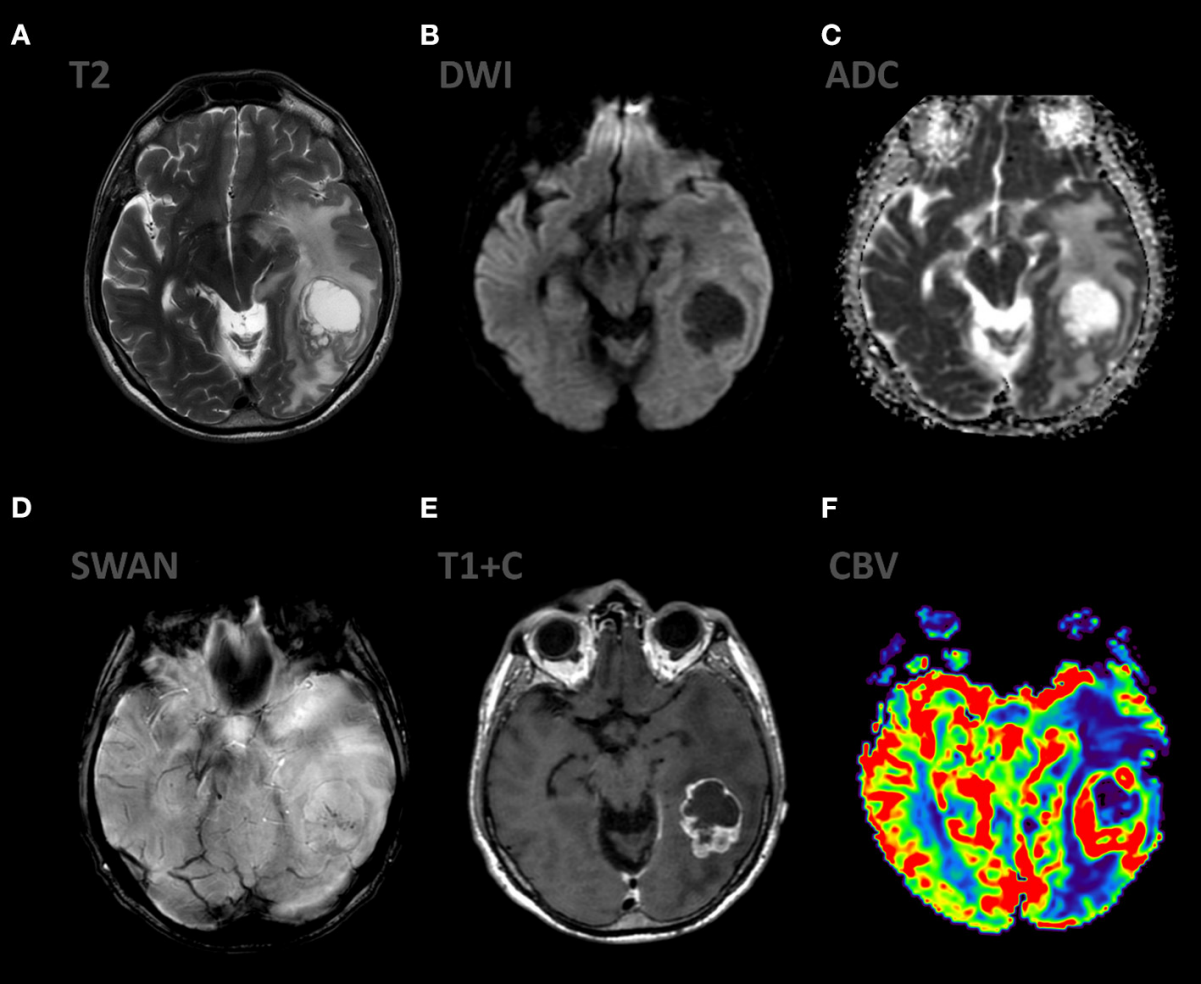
mpMRI ax: (A) T2WI; 6, (B) DWI, (C) ADC, (D) SWAN, (E) T1 + C, (F) DSC-PWI show classic appereance of solitary brain metastasis. There is a lesion with ring-like enhancing pattern and strong peripheral T2-hyperintensiry. Vasogenic edema is out of proportion with tumor size. The enhancing component of the lesion demonstrates high rCBV values.
